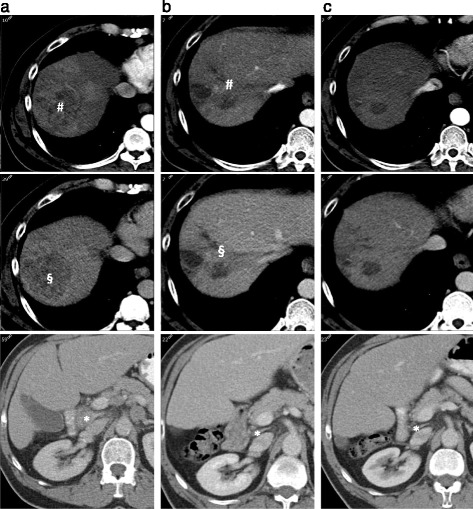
Case 1 response to sorafenib. a. Triple phase CT of the liver 07/12/2012. Primary HCC with arterial phase enhancement (# *top panel*) and portal venous washout (§ *middle panel*) with involved lymph node between portal vein and IVC (*bottom panel* *). b. Triple phase CT of the liver 03/04/2013. Primary HCC with reduced arterial phase enhancement (# *top panel*) and portal venous washout (§ *middle panel*) with two cystic areas. Reduction in size of lymph node (*bottom panel* *). c. Triple phase CT of the liver 15/07/2013. No arterial phase enhancement (*top panel*) or portal phase washout (*middle panel*) reflecting disappearance of tumour. Residual cystic areas. Sustained reduction in size of lymph node (*bottom panel* *)
